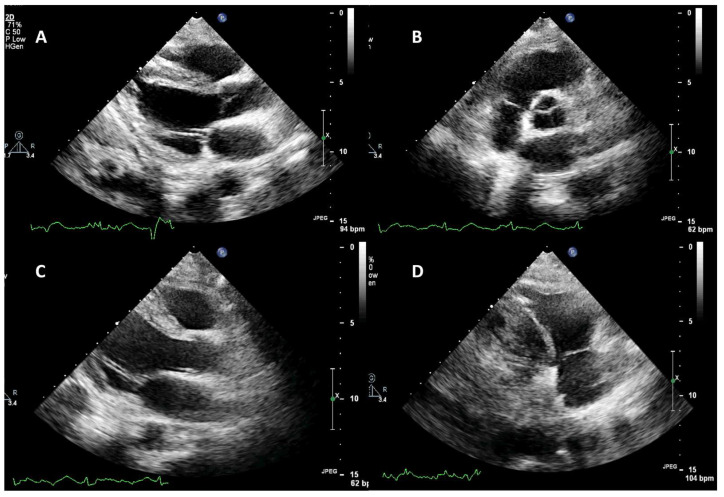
Echocardiographic evaluation of cardiac valves on the 20th ICU day, after the patient suffered the embolic episodes. (A,B) Parasternal long-axis and short-axis view of the aortic valve. There is no residual pathology indicating the initial vegetation. (A,C) Parasternal long-axis view of the mitral valve. No pathology is identified. (D) Modified parasternal long-axis view focusing on the right chambers. There is no lesion attached to the tricuspid valve.
